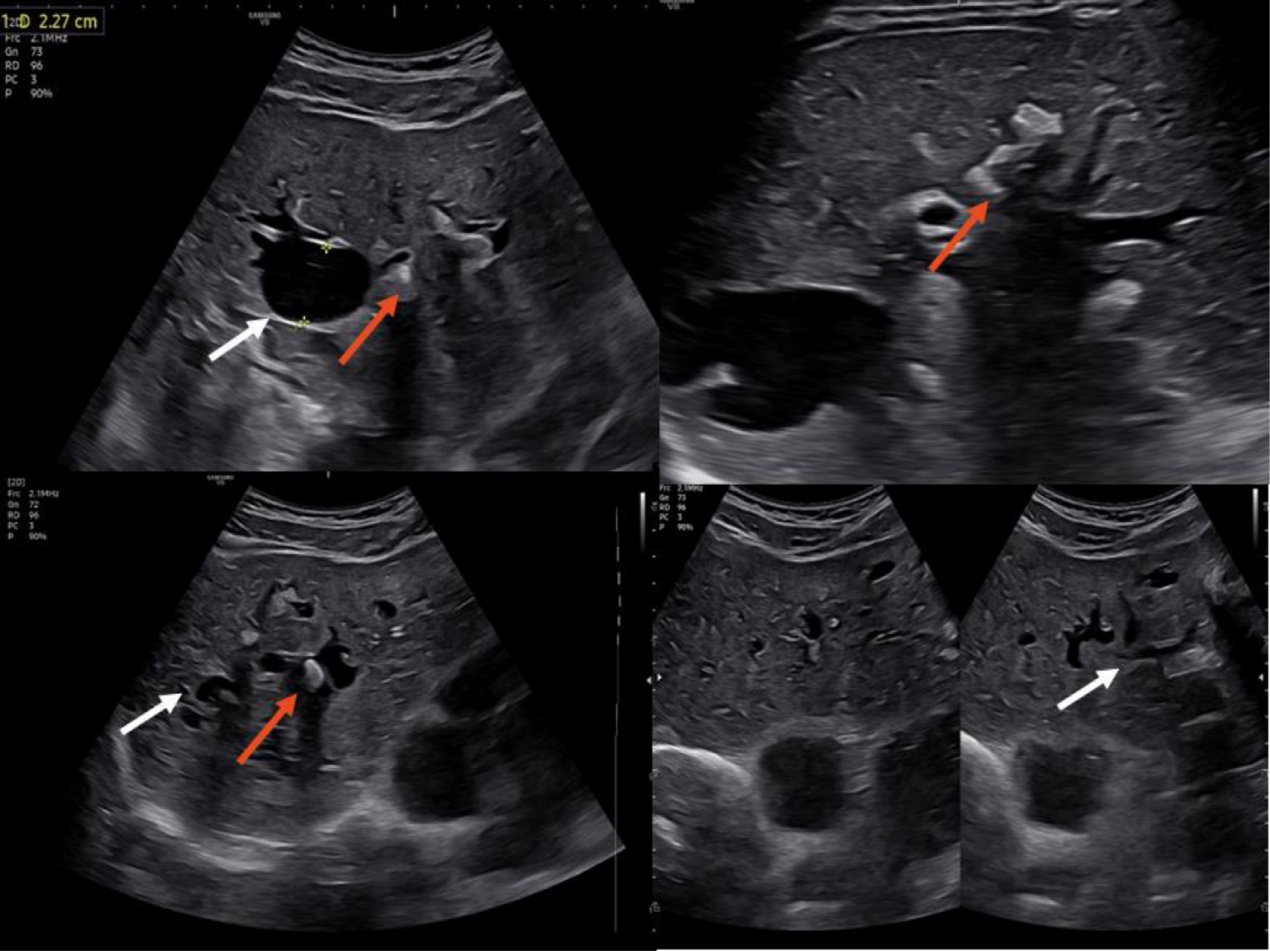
Abdominal ultrasound showing cystic dilation of the intrahepatic bile ducts (white arrows), with multiple stones inside (red arrows).
